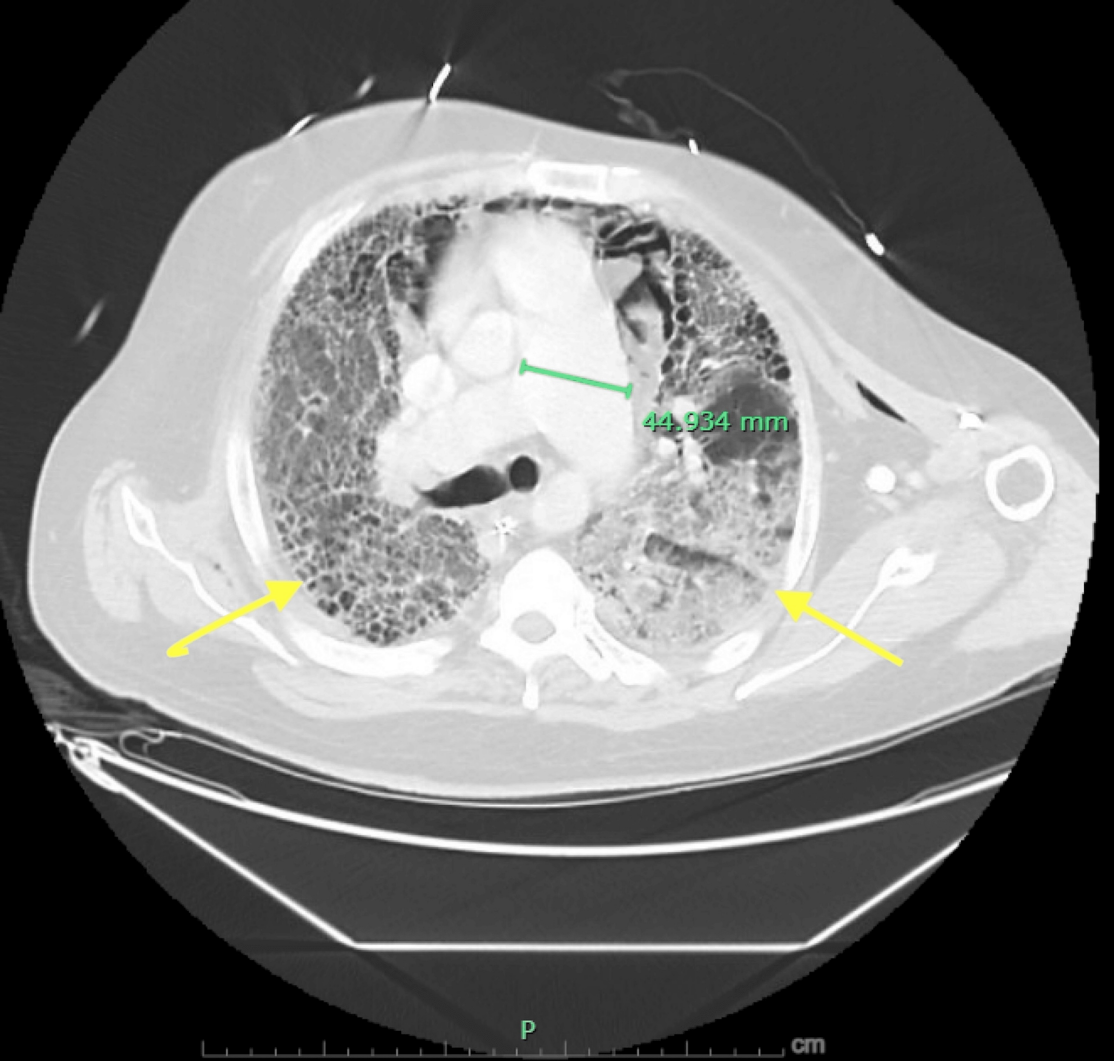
CT of the chest demonstrating extensive bilateral ground-glass opacities. The image demonstrates extensive bilateral ground-glass opacities (yellow arrows) with honeycombing. Note dilated main pulmonary artery measuring 44.9 mm, suggestive of pulmonary hypertension. CT: computed tomography.
